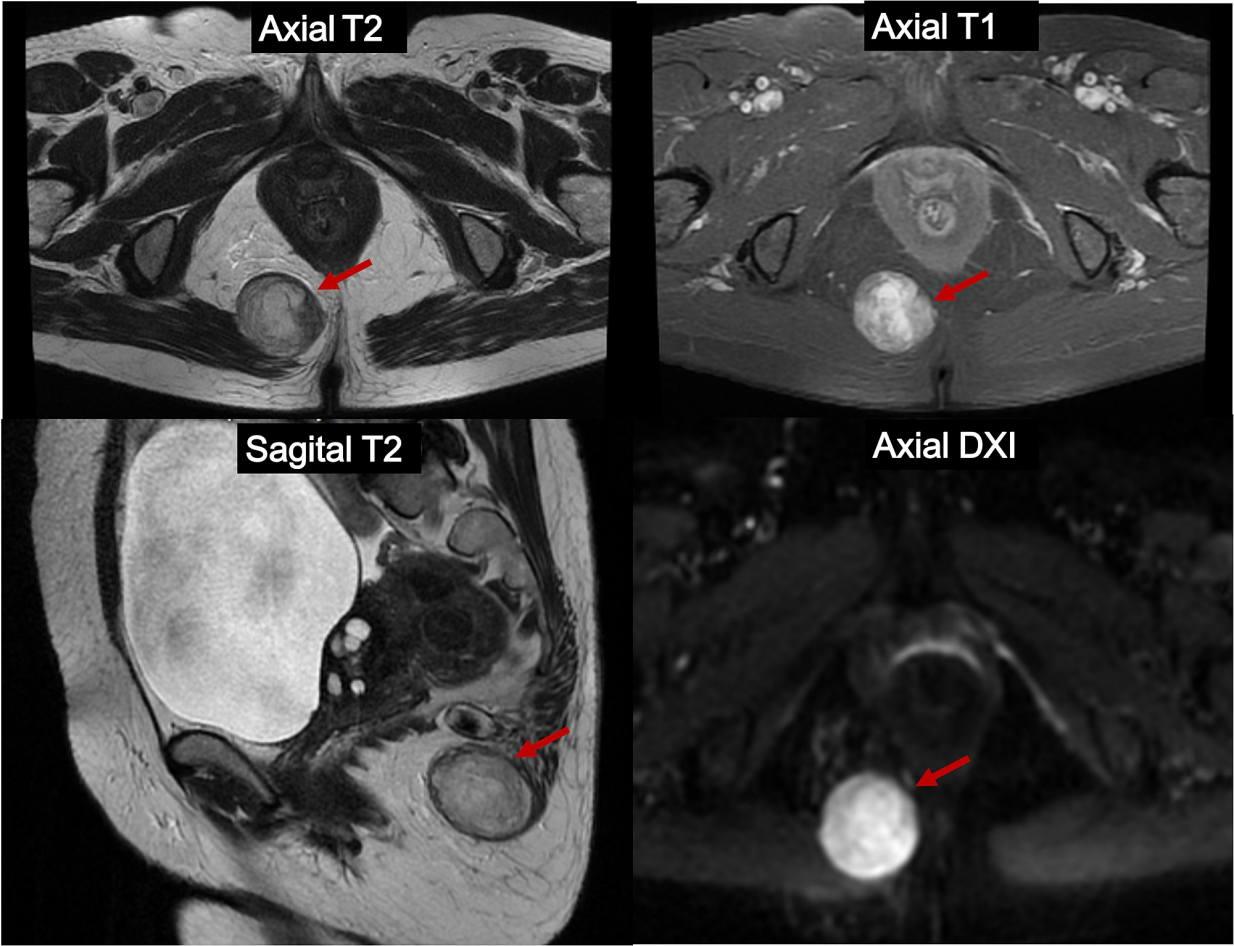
Contrasted MRI Well-defined oval lesion measuring 24 x 35 x 37 mm in the right ischiorectal fossa (Red arrow)
